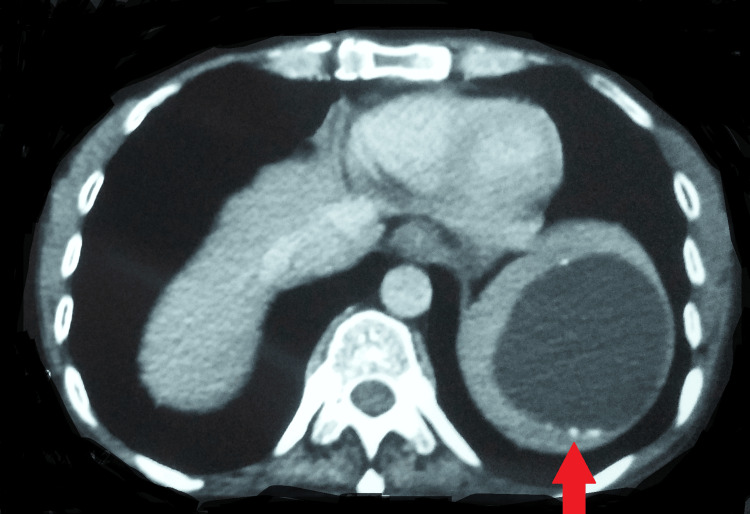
Contrast-enhanced computed tomography showing a well-defined cyst in the spleen
